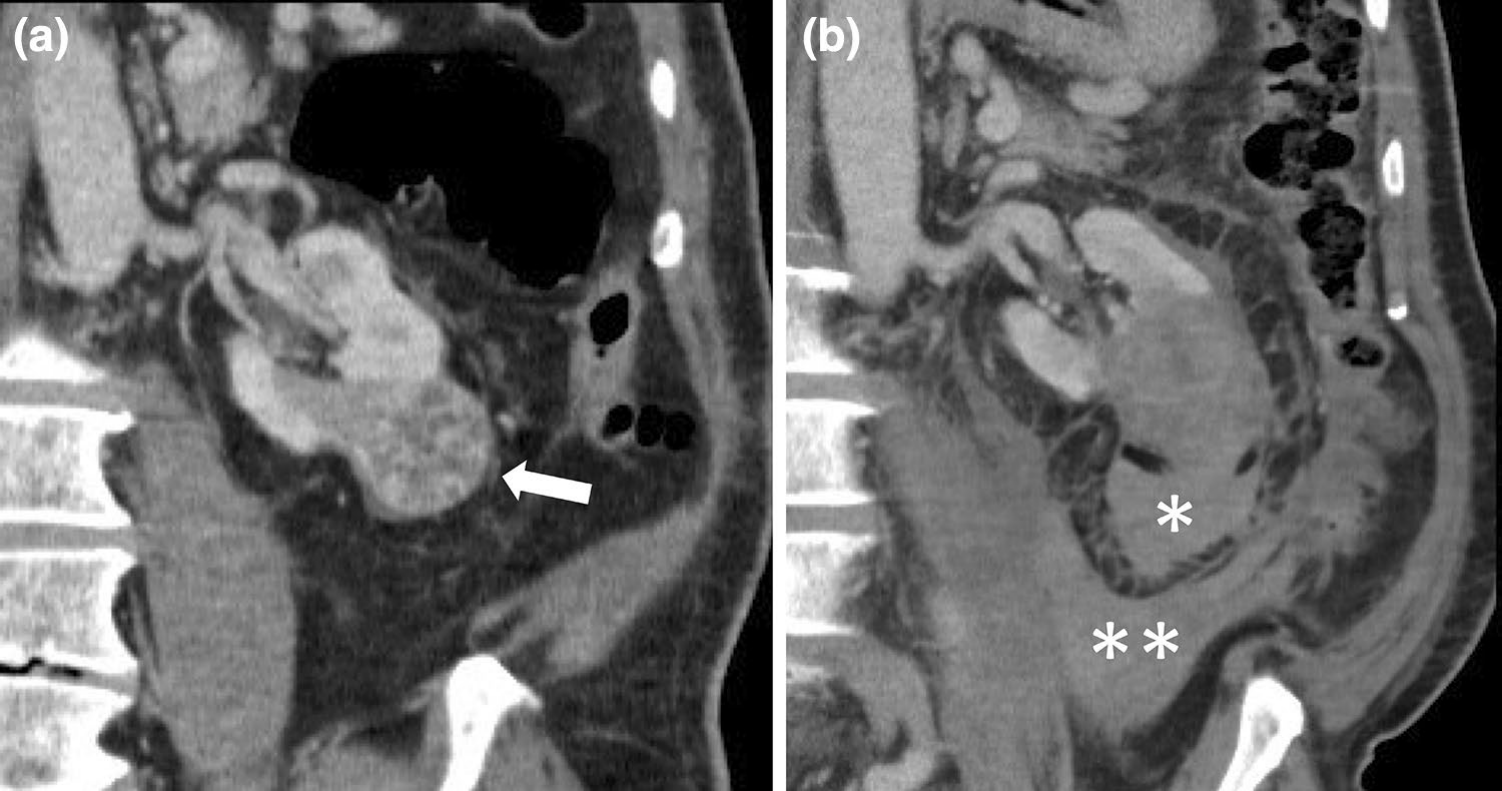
Hemorrhage after cryoablation of a RCC. a Pre-ablation CE CT shows a 3.5 cm enhancing mass at the lower pole of the left kidney (arrow). b CE CT obtained 1 day after cryoablation shows a perirenal hematoma (*) adjacent to the ablated area together with a pararenal hematoma (). The patient recovered following transfusion
